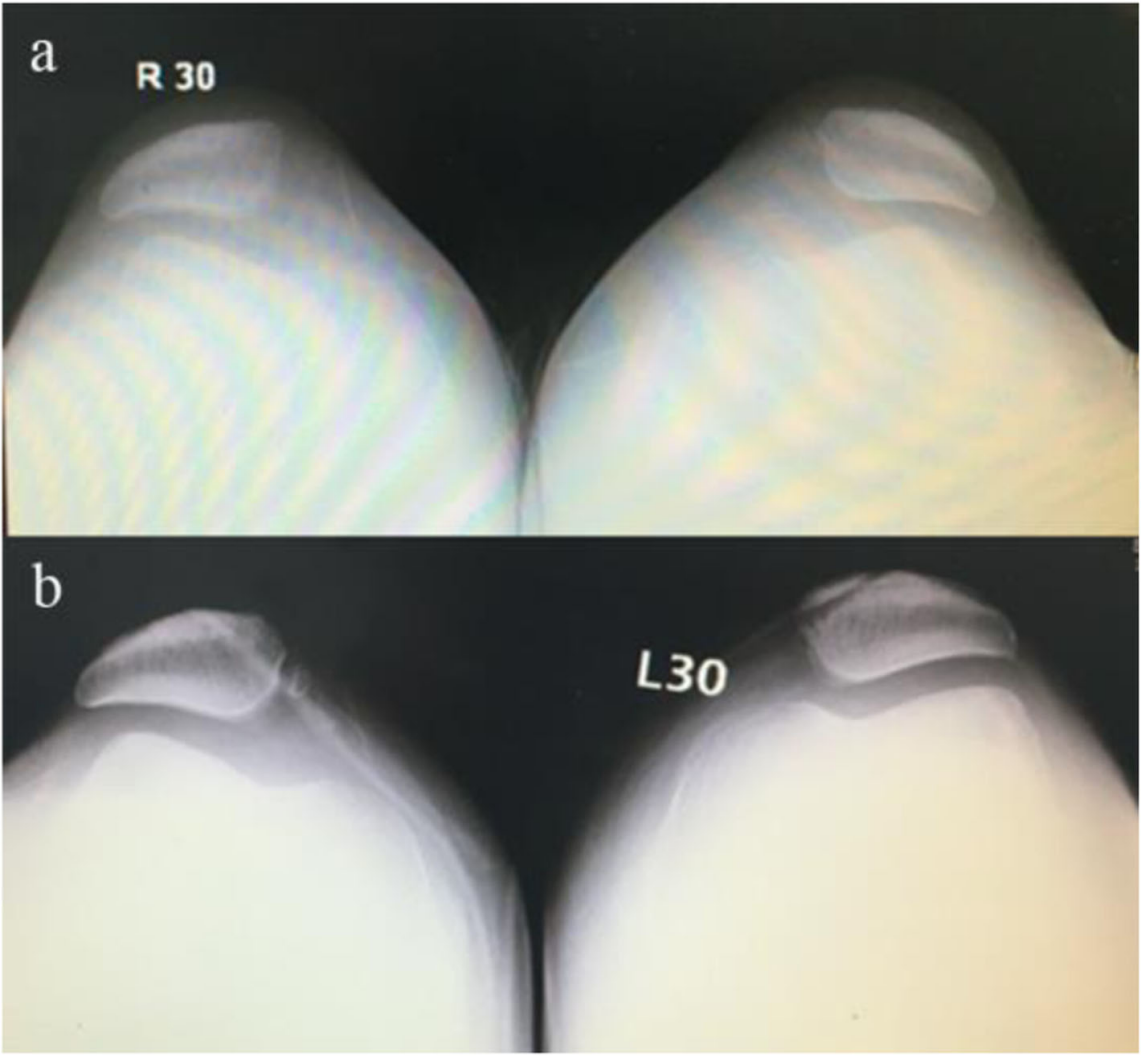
Comparison of preoperative (a) and postoperative (b) Merchant-view radiographs demonstrating restoration of patellofemoral tracking
